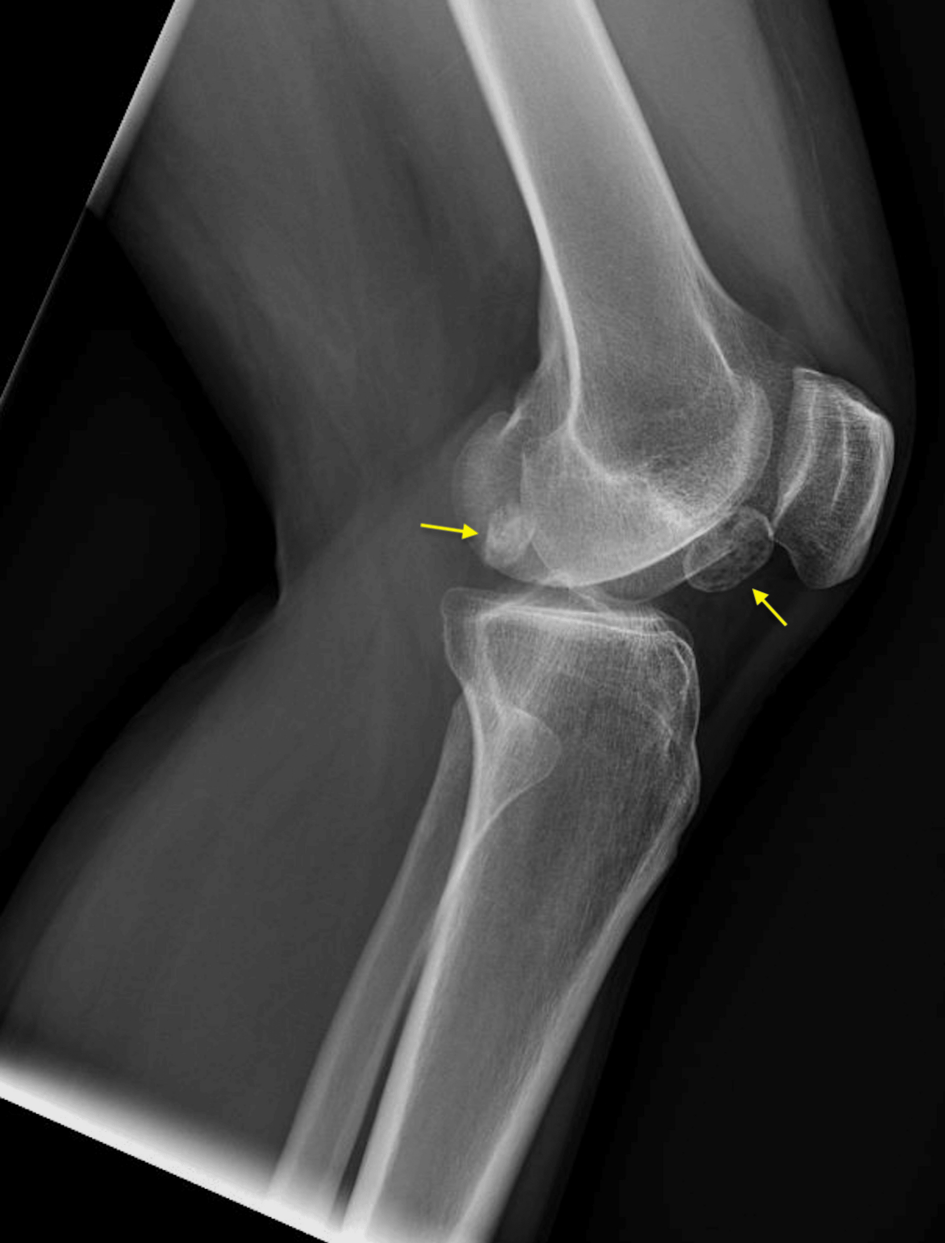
X-ray of the left knee (lateral view) showing multiple intra-articular loose bodies (yellow arrows).
